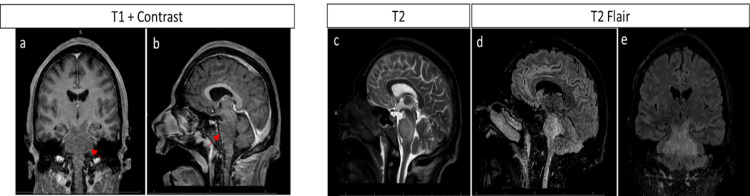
MRI Brain with contrast on day four of receiving steroids showing expansion of the lesion to bilateral pons, cerebellar peduncles and touching mildly the cerebellum. Notice that the enhancement of the lesion and the edema had faded after receiving steroids (a) +(b) (red arrow). (c), (d) and (e) are showing the expanded lesion on T2 and T2 FLAIR imaging. FLAIR: Fluid-Attenuated Inversion Recovery.
